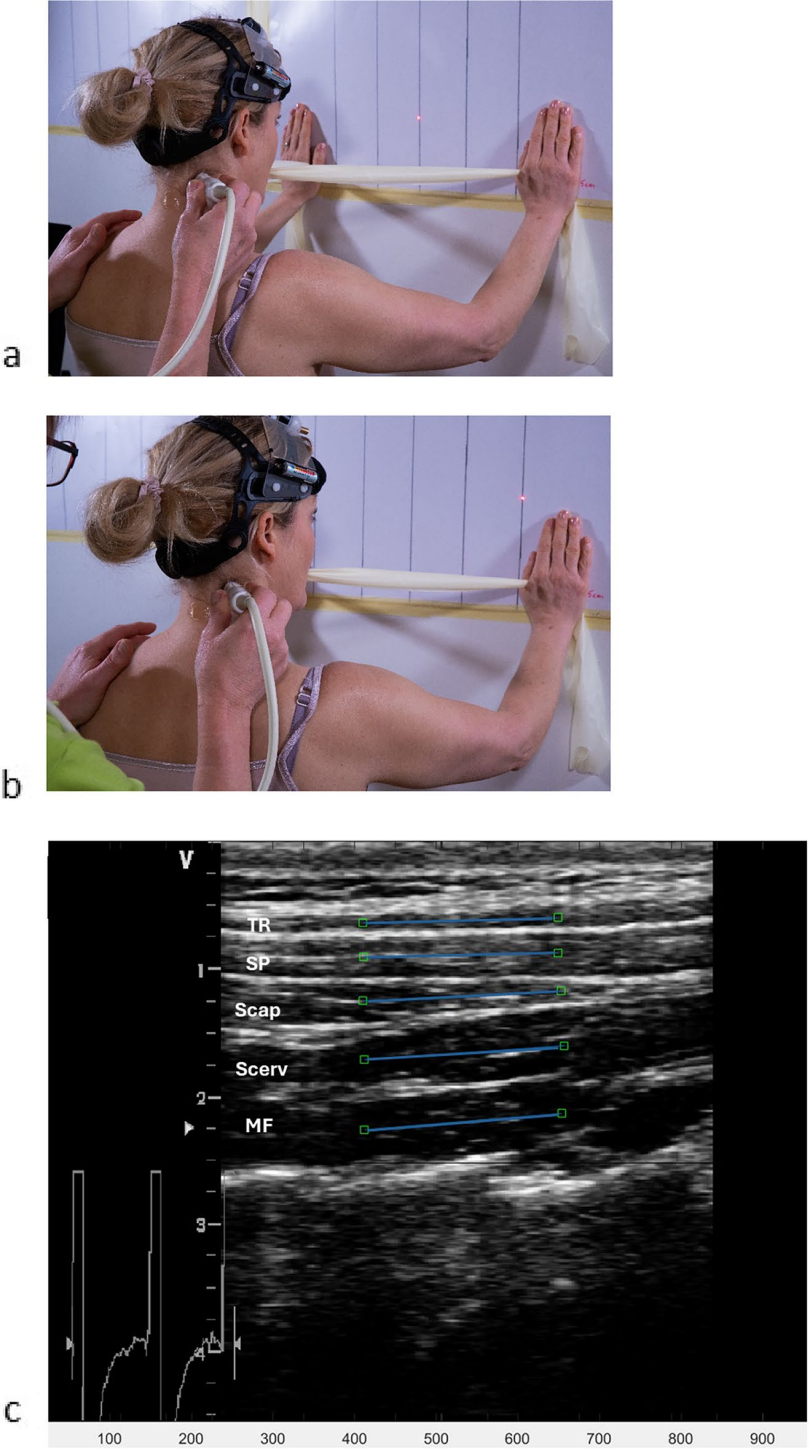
(a) Starting position for the rotation test. Five lines were marked on the wall: starting position (midline), two lines to the right (first line 10-degree rotation, second line 20-degree rotation), and two lines to the left (first line 10 degrees, second line 20 degrees). A self-chosen neutral head position was the starting position. The laser cursor at the helmet was adjusted to the starting line (midline marked on the wall). The participant held a rubber band with light resistance (TheraBand Tan) gently between their teeth in line with their mouth. The rationale for using the task in the present study was to investigate if resistance neck rotation could detect altered neck muscle function in chronic WAD and be used to evaluate muscle function after an exercise programme. In our clinical experience, light resistant neck rotation improves neck pain and disability in many chronic WAD patients, indicating that this exercise improves neck muscle function which may be important for good outcomes. (b) Twenty-degree rotation to the right. (c) The ultrasound image shows the longitudinal projection with the superficial trapezius (TR) at the top, followed by splenius (SP), semispinalis capitis (Scap), semispinalis cervicis (Scerv), and multifidus (MF). A region of interest (ROI) was manually placed in the first frame of the video sequence of the muscle, measuring the deformation (elongation and shortening) and the deformation rate (how fast the shortening and elongation occur) using speckle-tracking analyses. One 15 mm ROI was placed in each muscle (indicated as a blue line with a square at each end).
